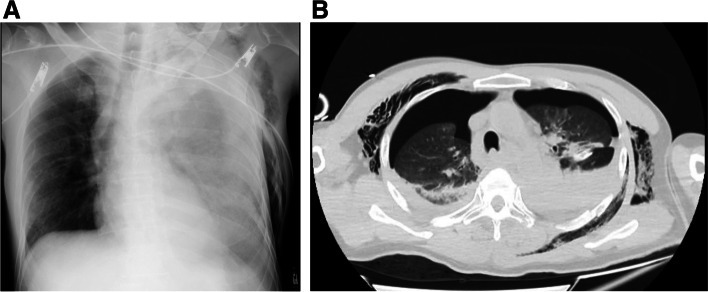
A A hemopneumothorax was found in the left thoracic cavity on admission chest radiography. B Chest computed tomography on admission revealed bilateral hemopneumothorax
